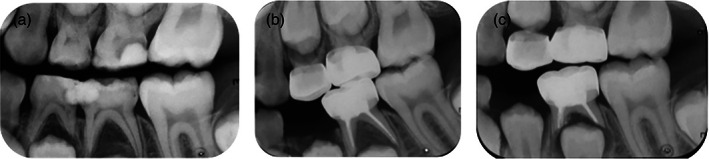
Successful Vitapex pulpectomy treatment in a lower second molar with deep carious lesion. (a) Pre‐operative baseline radiograph. (b) Six‐months follow‐up radiograph showing reduction in the size of the bifurcation radiolucency. (c) Twelve‐months follow‐up
